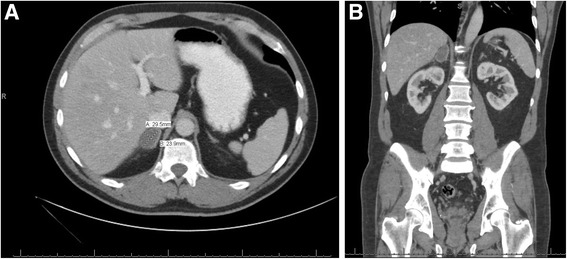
a One-month follow-up computed tomography scan. The right adrenal gland hemorrhage had improved and reduced to 3.0 × 2.4 cm in diameter. The previously noted right periadrenal fatty stranding was almost completely resolved. b Coronal view of 1-month follow-up
